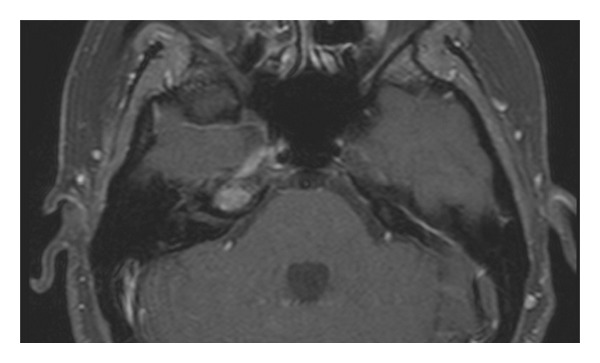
Axial T1-weighted image with Gadolinium showes the absence of postcontrast enhancement in the lesion of the right petrous apex.
